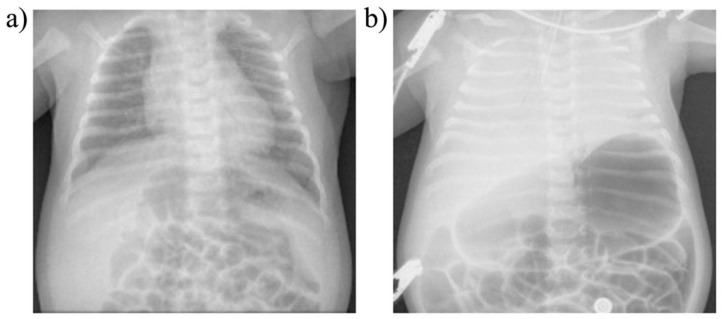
Rapid interval chest X-ray findings in an infant with terminal RSV bronchiolitis. (a) Normal-appearing CXR with some streaky lung infiltrates. (b) Marked changes 2 h later after sudden respiratory distress, with complete bilateral lung field opacification and ectasia of the stomach and proximal bowels. The opacity is probably from bilateral fluid collections (thought to occur by DCC airway obstruction). Creative Commons Attribution 4.0 International License. Source: Bottino et al. [168].
